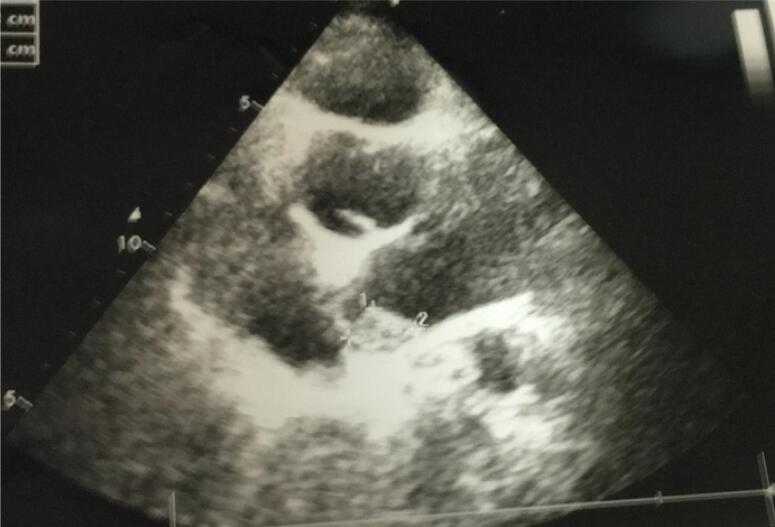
Echocardiography showed a 2.1 by 1.8 cm myxoma in base of left atrium.
